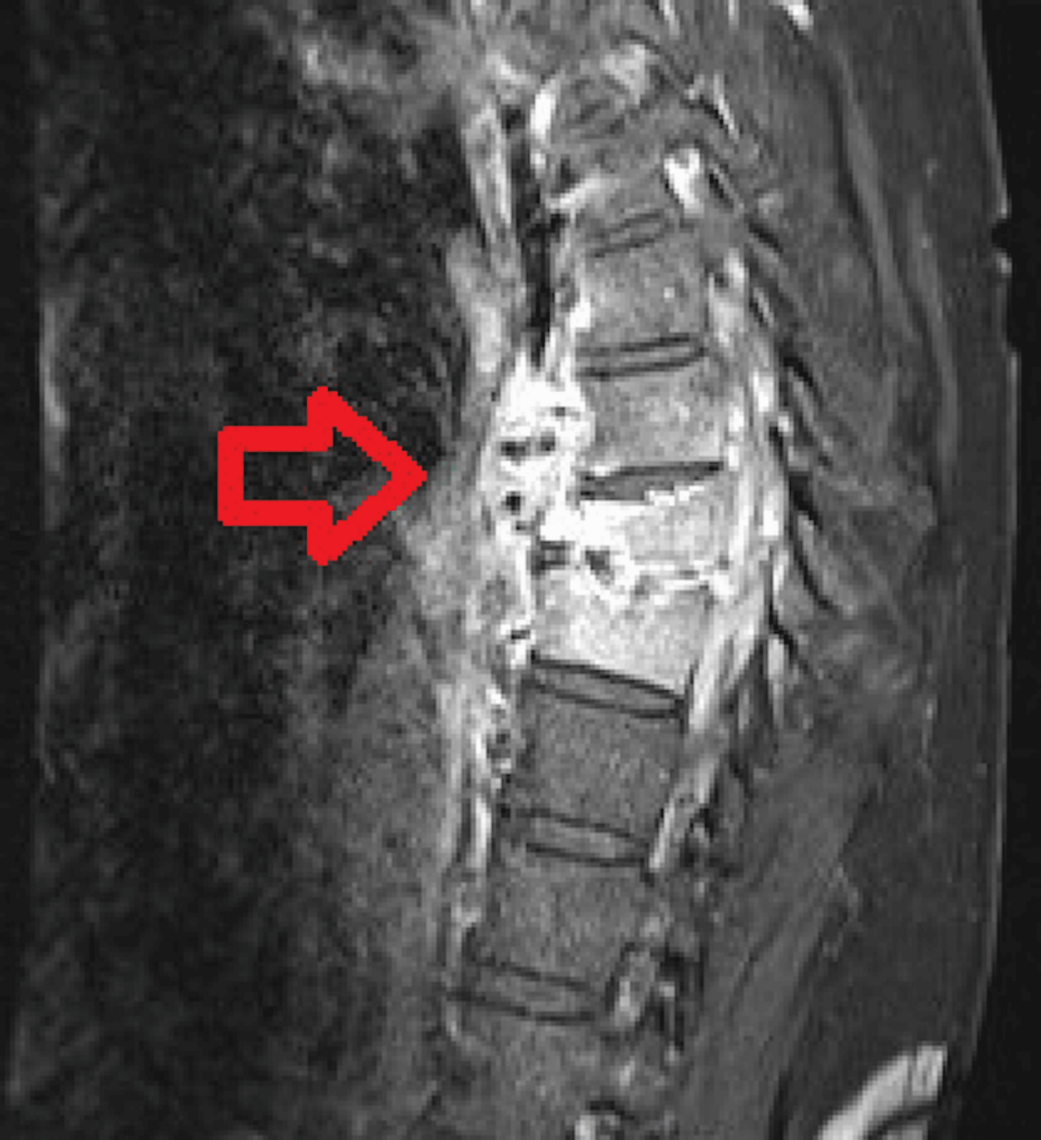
MRI acquired the day before surgery.
